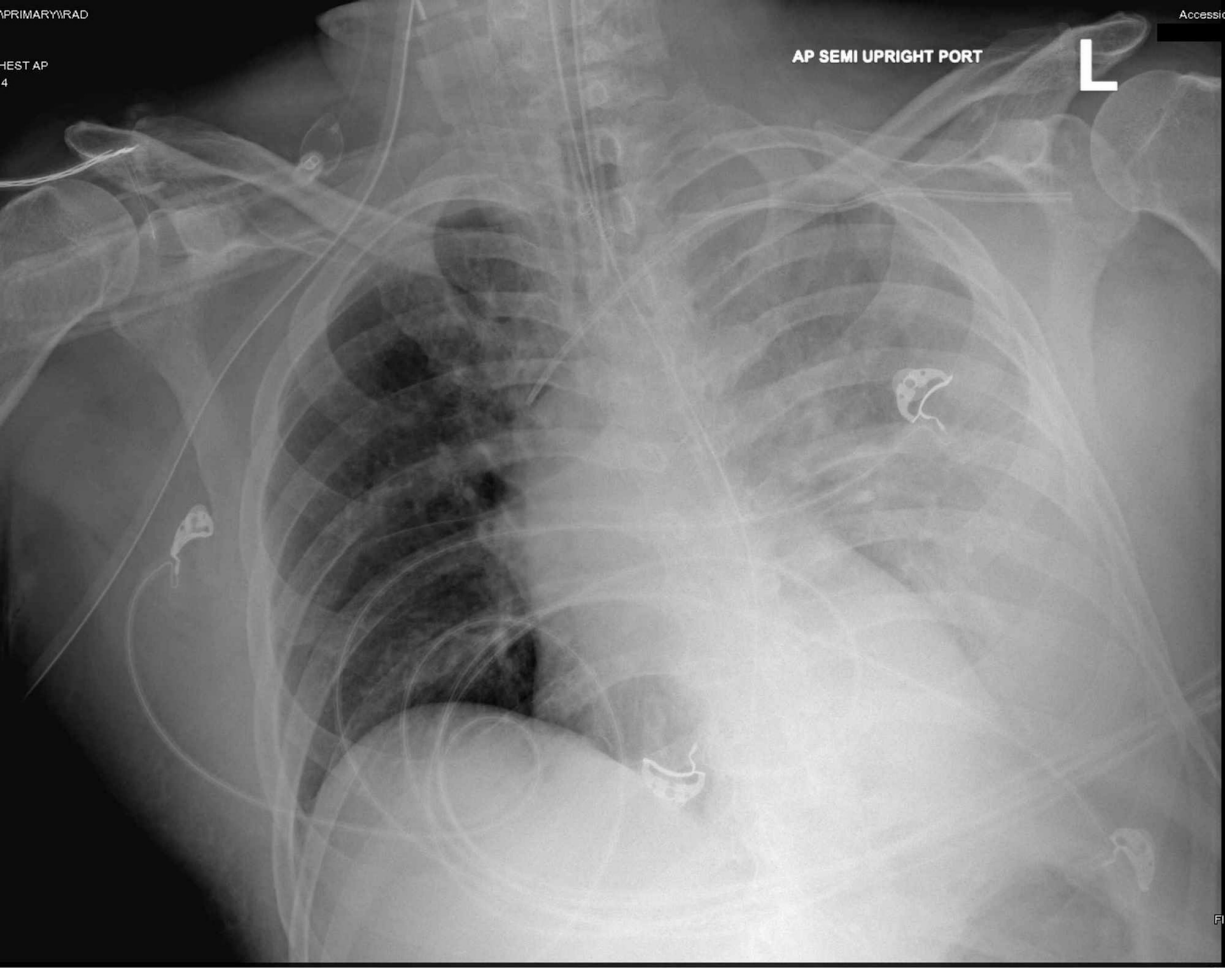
Chest X-ray showing worsening left lung opacity
